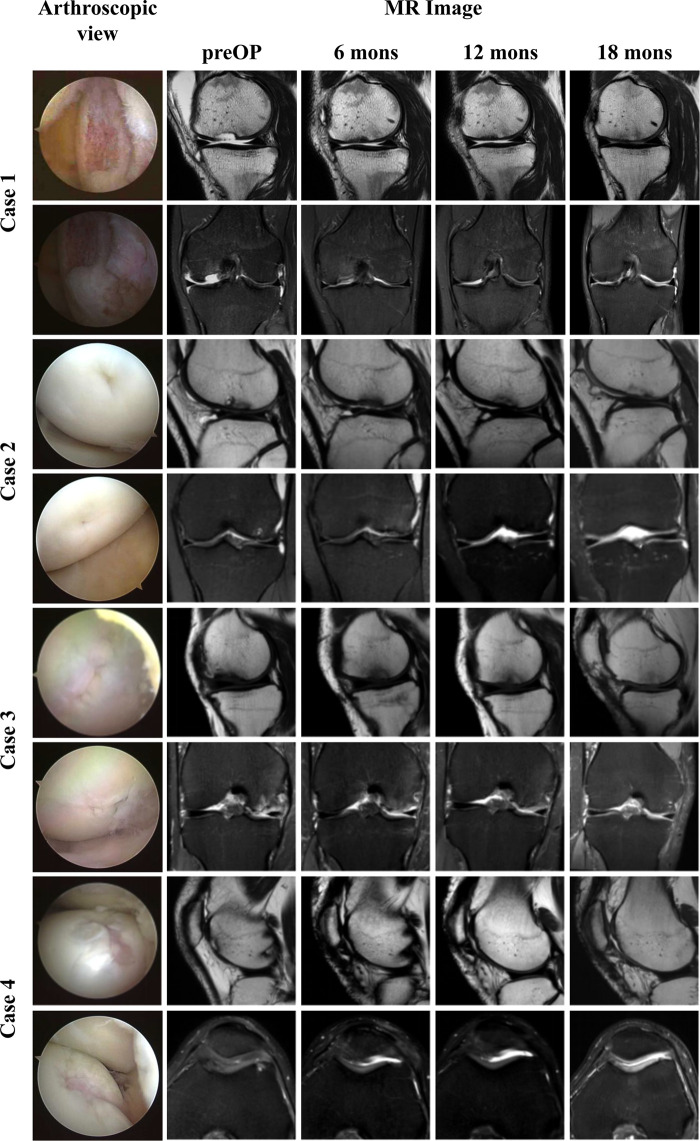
Arthroscopic view and MR images of four patients. The first column: arthroscopic view of cartilage defect. The second to fifth columns: Sagittal T2-weighted, coronal, or axial PD-weighted MR images of four patients from pre to 18 months after procedure.
